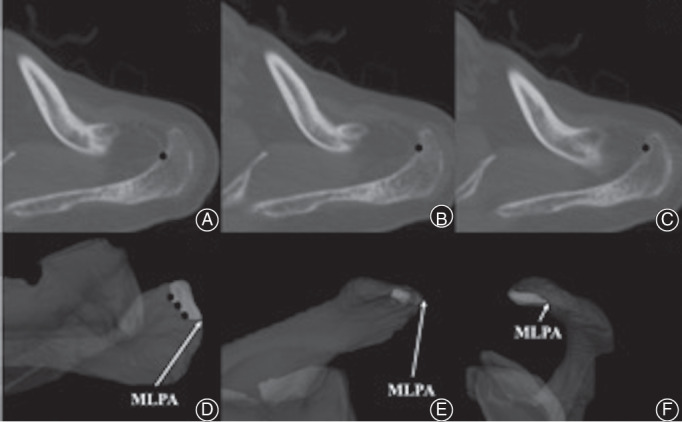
Localization of acromial anterolateral protrusion (AALP). (A), (B), (C) The inflection point (black dots) with the greatest curvature of the anterolateral curve was marked along the inner edge of the acromion on the CT image of the anterior half of the acromion. (D) Position of the point marked on the CT image in the x–z plane of the three‐dimensional model. The edge formed by the black dots were the inner edge of the AALP (dark white area). (E) x–y plane view of AALP. (F) y–z plane view of AALP. MLPA, most lateral point of acromion.
